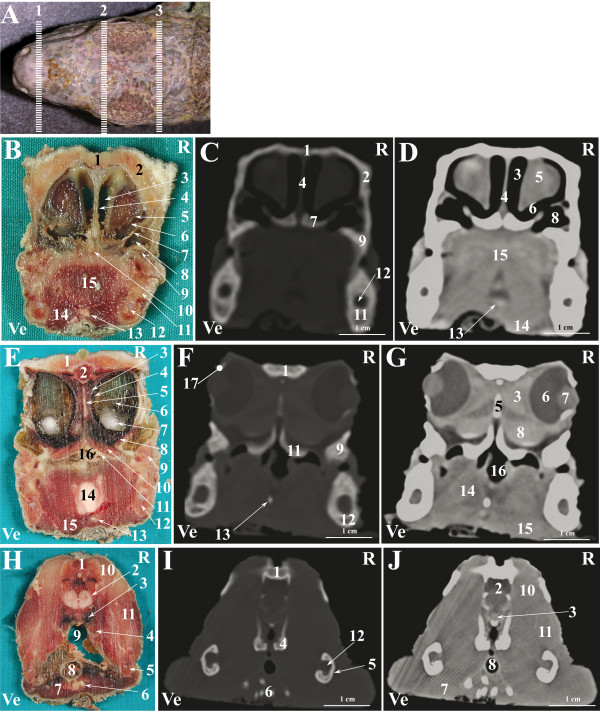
Normal computed tomographic anatomy of the head of the green iguana.A. Photograph of the head of an iguana; lines (1–3) indicate the approximate levels of matched cross-sections and CT images. B- J: Matched images of cross-sectional gross anatomy ( B- E- H), CT image displayed in bone window (WW: +2000; WL: +500) ( C- F- I) and CT image displayed in soft tissue window (WW: +400; WL: +40)( D- G- J) corresponding to: line 1 ( B- C- D), line 2 ( E- F- G) and line 3 ( H- I- J) as depicted in Figure 3A. R is right and Ve is ventral. B- C- D: 1. Nasal bone; 2. Prefrontal bone; 3. Stammteil; 4. Nasal septum; 5. Nasal glands; 6. Subconchal recess; 7.Vomer bone; 8. Choanal tube; 9. Maxillary bone; 10. Cavum oris; 11. Dentary bone; 12. Meckelian fossa; 13. Entoglossal process of the basihyal bone; 14. Musculus geniohyoideus + Musculus hyoglossus + Musculus intermandibularis; 15. Tongue. E- F- G: 1. Frontal bone; 2. Brain; 3. Harderian gland; 4. Choroid plexus; 5. Interorbital septum; 6. Vitreous humor; 7. Lens; 8. Sinus orbitalis; 9. Maxillary bone; 10. Palatine glands; 11. Palatine bone; 12. Dentary bone; 13: Entoglossal process of the basihyal bone; 14. Tongue; 15. Musculus geniohyoideus + Musculus hyoglossus + Musculus intermandibularis; 16. Cavum oris; 17. Scleral ossicles. H- I- J: 1. Parietal bone; 2. Brain; 3. Vena cerebralis media; 4. Sphenoid bone; 5. Angular bone + Surangular bone; 6. Hyobranchial skeleton; 7. Musculus intermandibularis + Musculus genioyhoideus + Musculus hyoglossus + Musculus pterygoideus + Musculus omohyoideus + Musculus stermohyoideus + Musculus ceratohyoideus; 8. Trachea; 9. Oesophagus; 10. Musculus adductor mandibulae externus medialis; 11. Musculus adductor mandibulae externus superficialis; 12. Adductor fossa.
